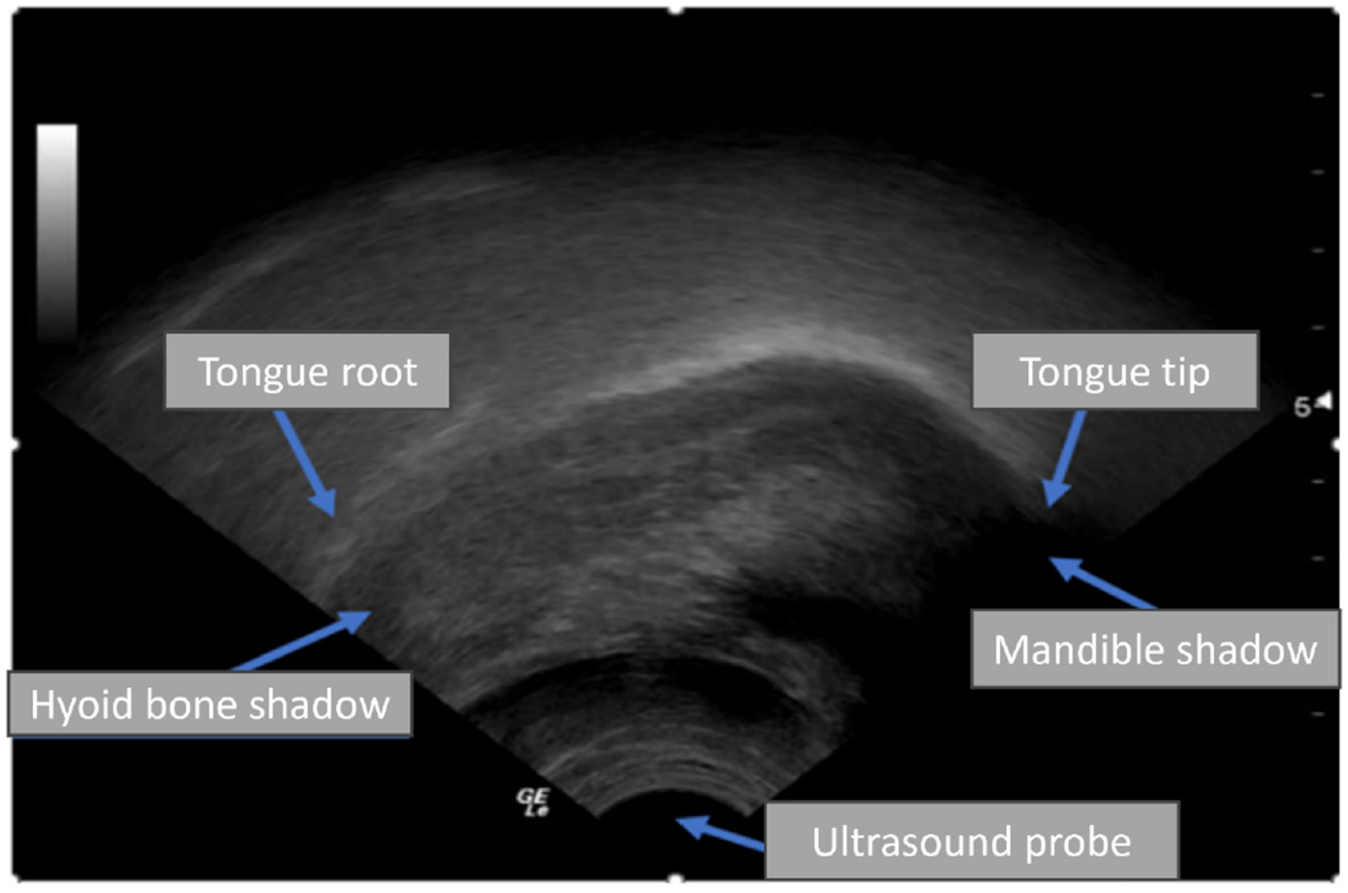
An ultrasound scan shows the tongue root and tip in the sagittal plane.
